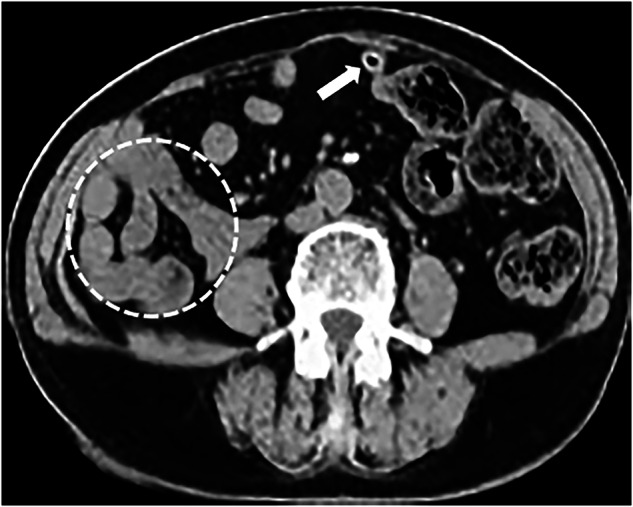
Sixty-year-old woman with midgut malrotation. Axial CT scan showed the jejunum located in the right abdomen (circle), while the ascending colon, cecum, and appendix (thick arrow) located in the left abdomen
